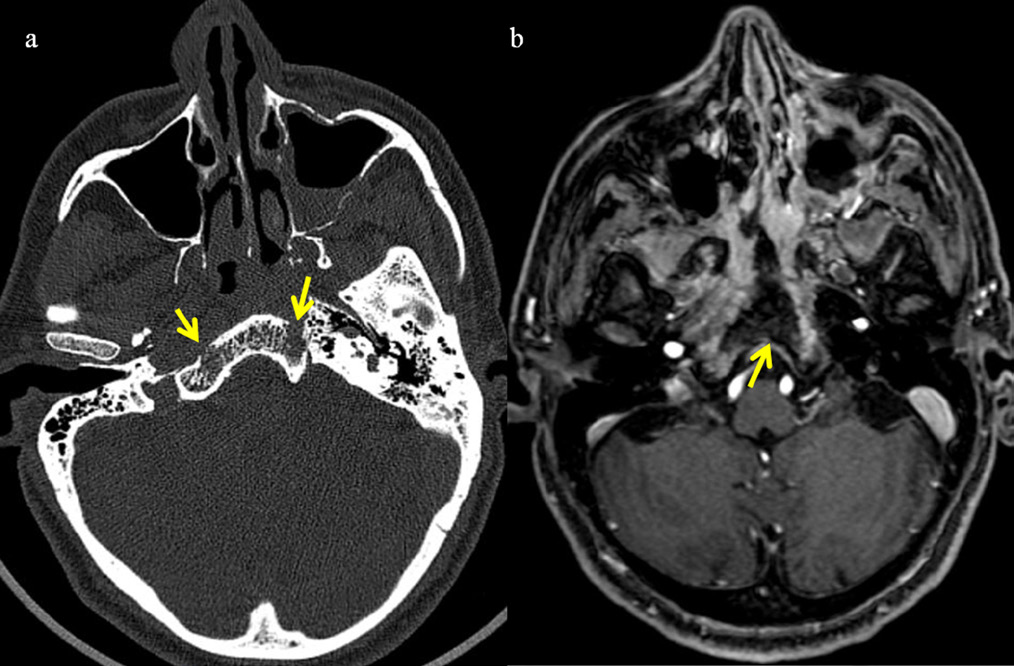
Axial bone window section of CT(a) and post-contrast axial T1FS MRI (b) showing bone invasion. (a) Permeative destruction noted involving the clivus (arrow). (b) Non-enhancing areas involving the clivus indicating necrosis (arrow).
